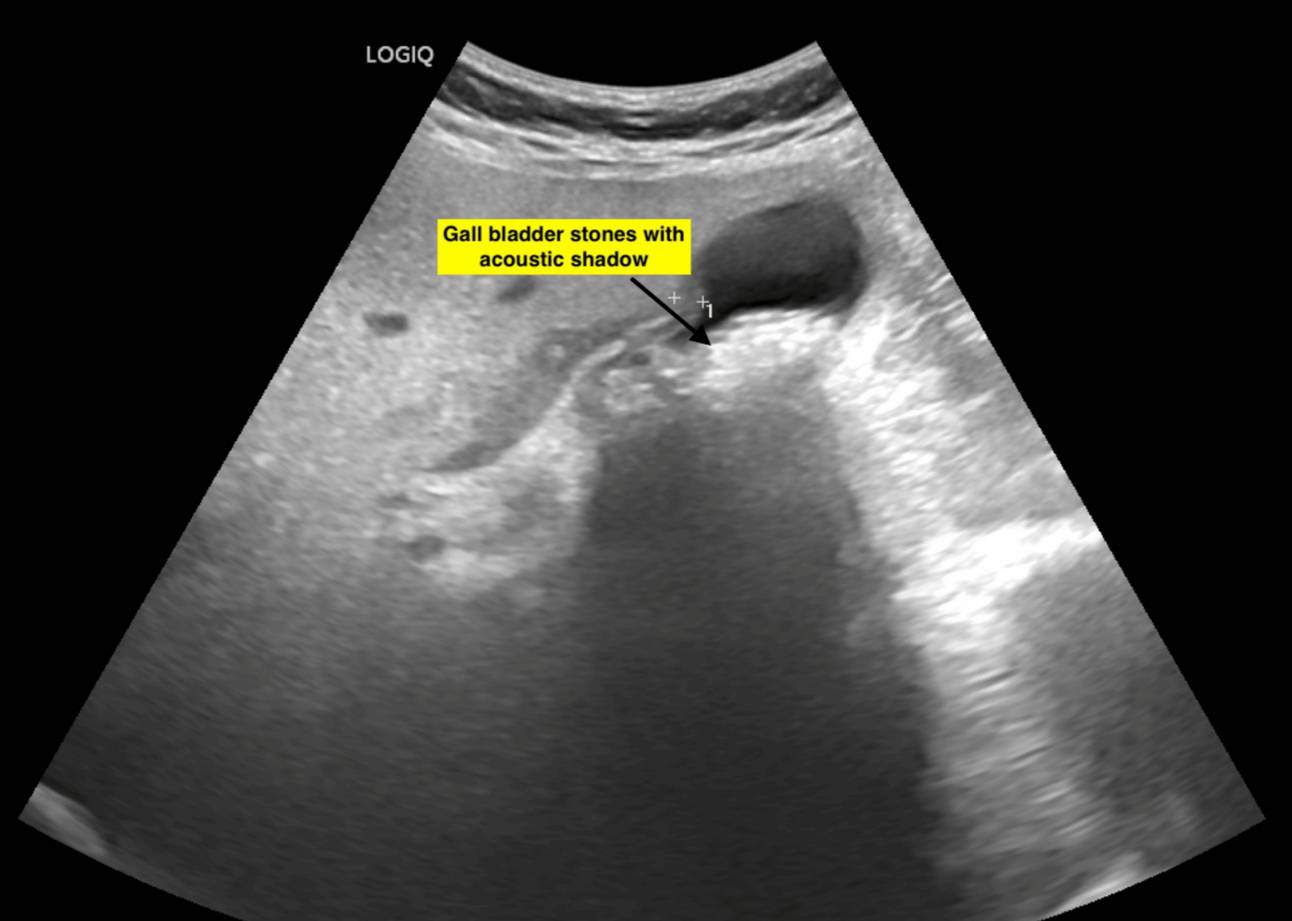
Ultrasound image showing gallbladder stones
